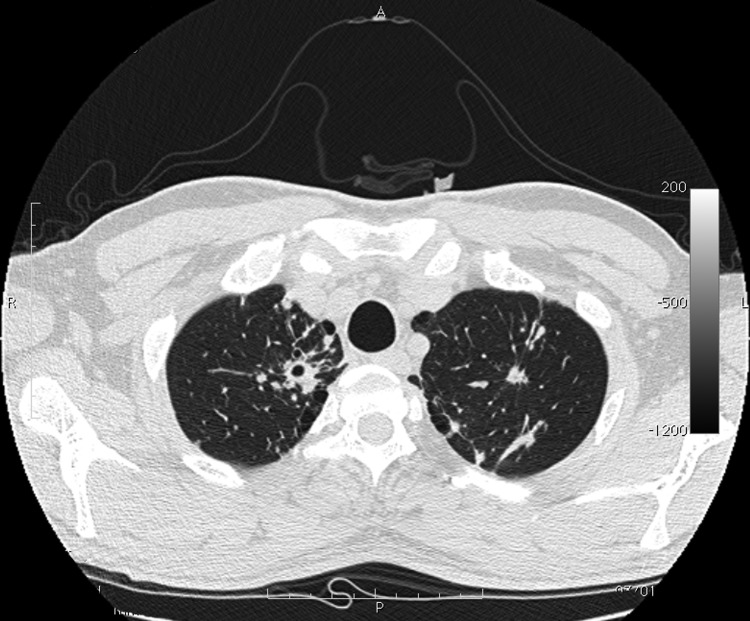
Computed tomography image of chest of patient with tuberculosis after anti–hepatitis C virus therapy. A parenchymal distortion 32 mm in diameter is shown in the upper right lung with initial central excavation 10 mm in diameter. Similar lesions 8 mm in diameter without central excavation are shown in the upper left lung.
